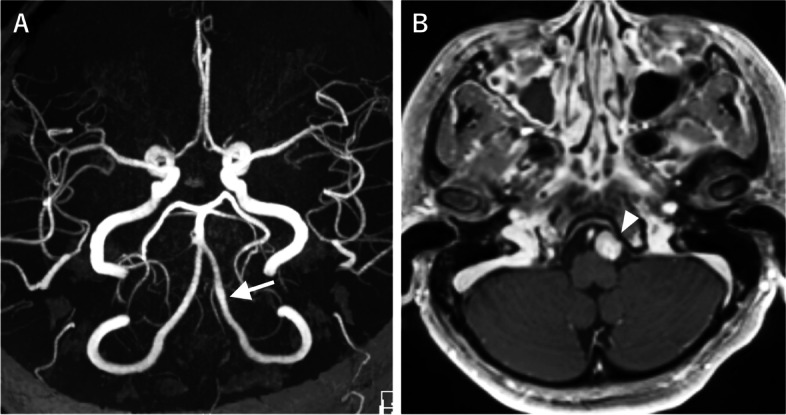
Magnetic resonance (MR) images of Case 2. A: Time-of-flight MR angiography revealing only minimal luminal irregularity in the left vertebral artery (arrow). B: Contrast-enhanced T1-weighted image revealing a lesion resembling a fusiform aneurysm in the left vertebral artery (arrowhead)
